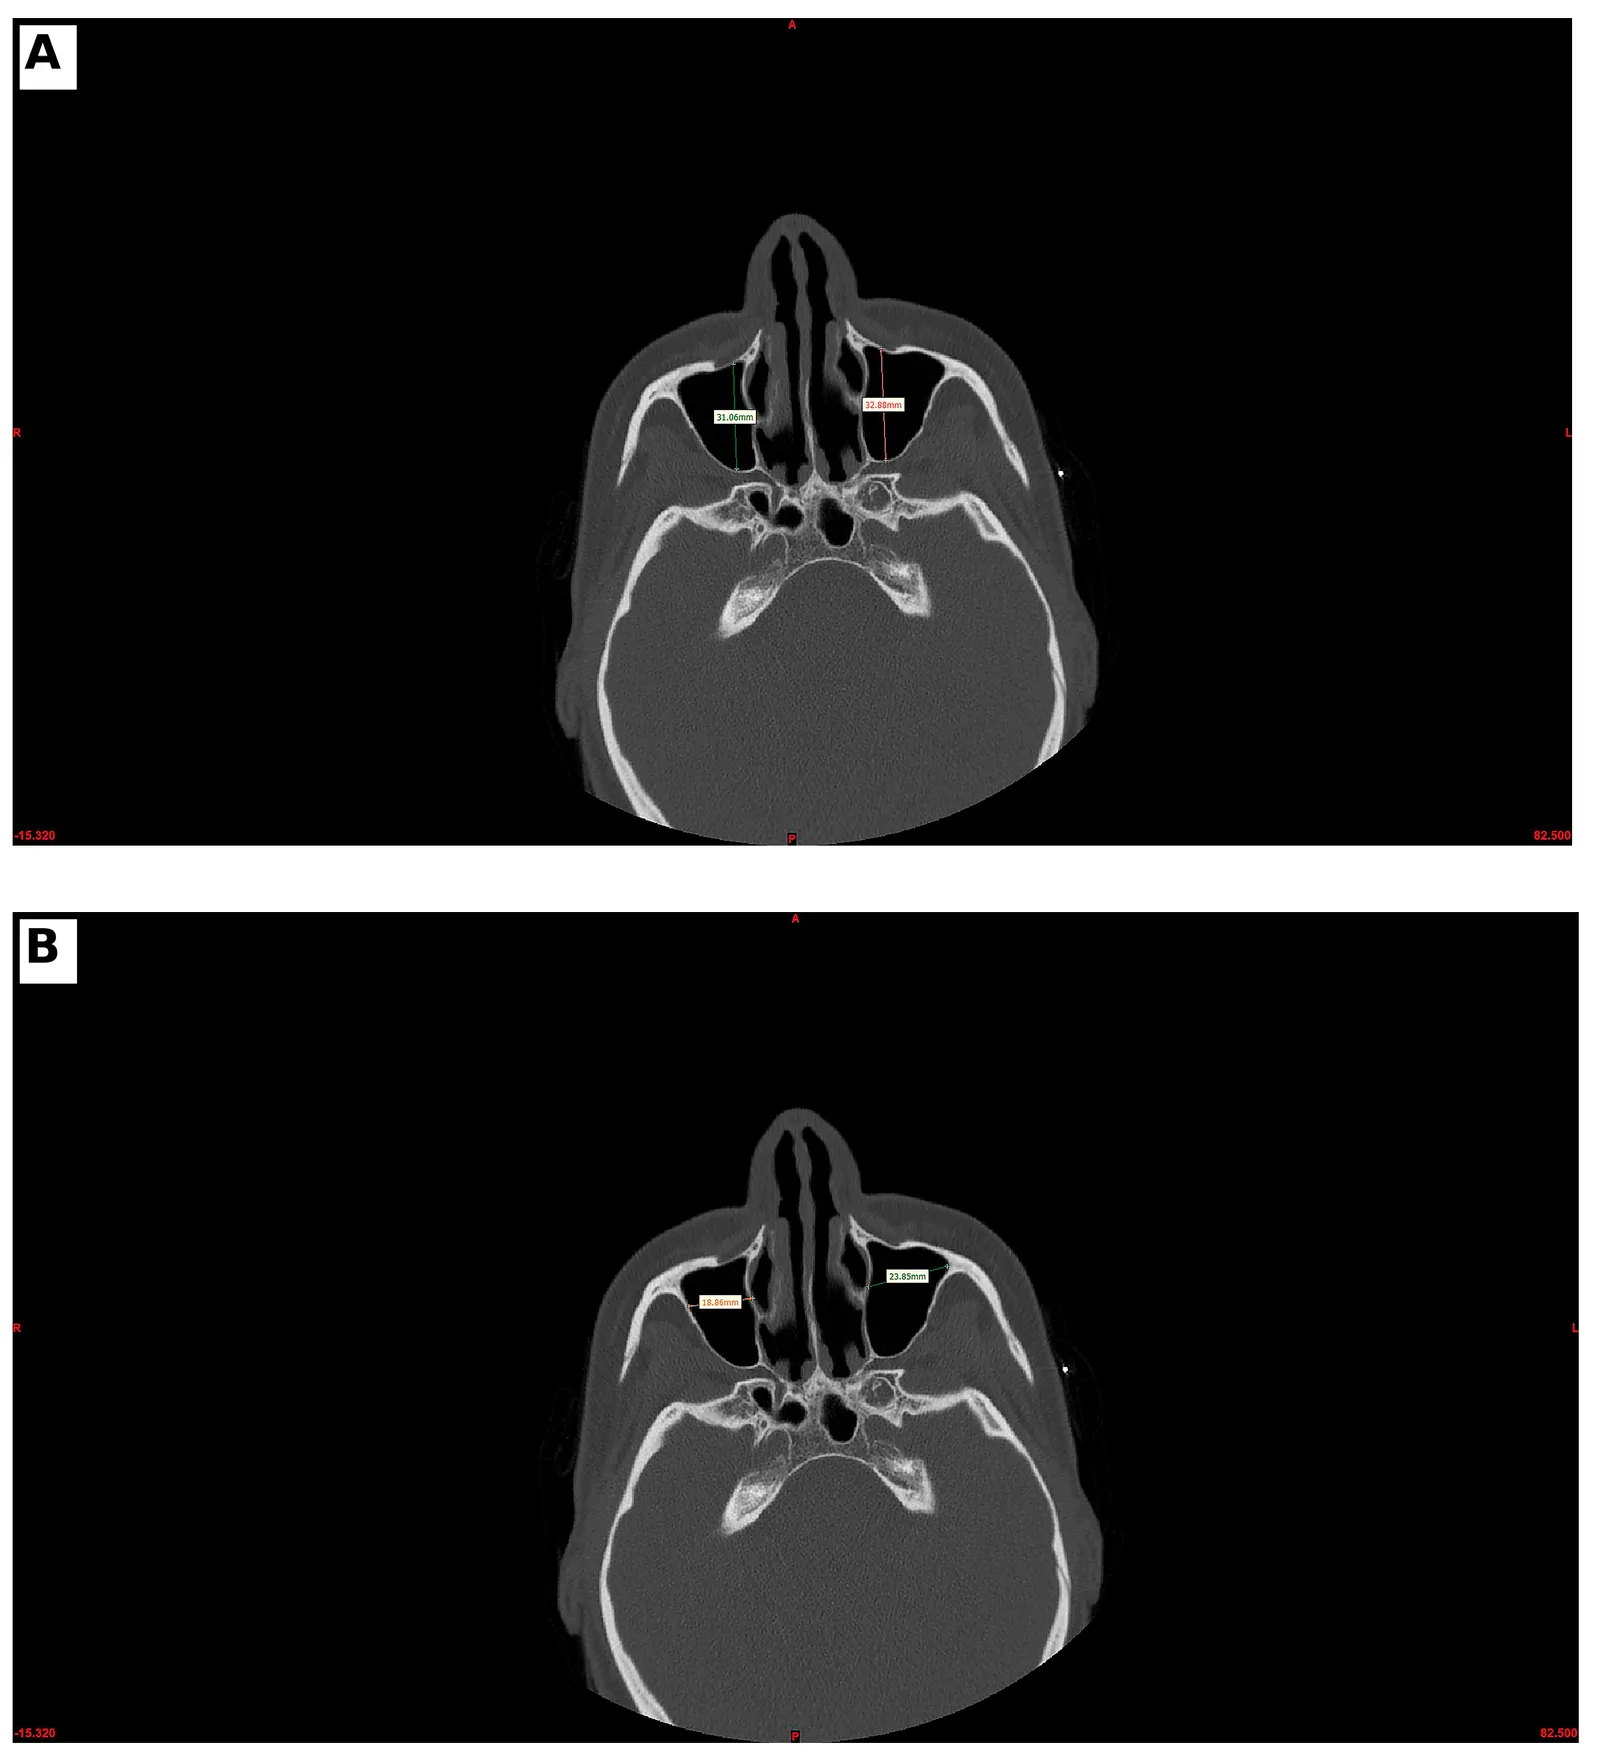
Axial morphometric measurements of the maxillary sinus. (A) Maximum anteroposterior diameter measured on the axial CT section. (B) Maximum transverse diameter measured on the axial section showing the largest cross-sectional area of the maxillary sinus. Abbreviations: A, anterior; P, posterior; R, right; L, left.
